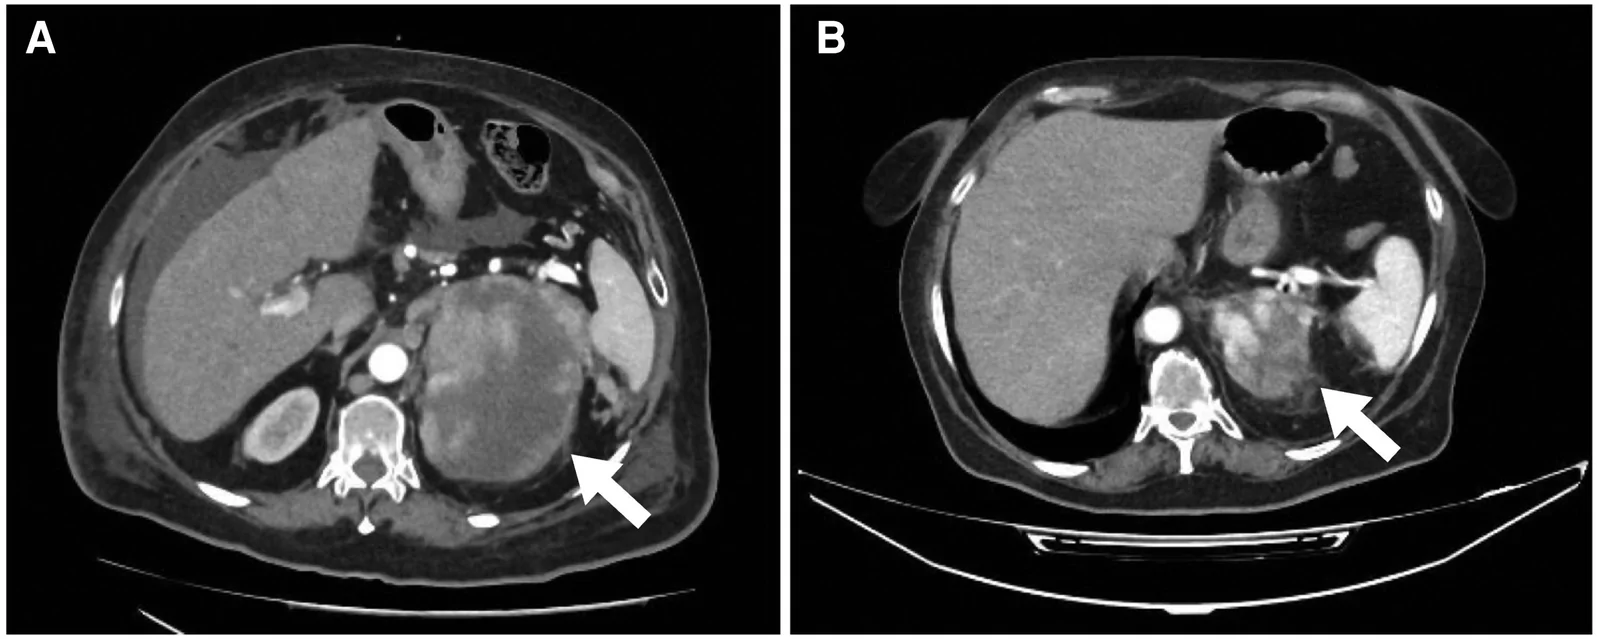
Comparison of left adrenal lesion size between the computed tomography scan of April 2023 (A) and the computed tomography scan from June 2023 (B).
